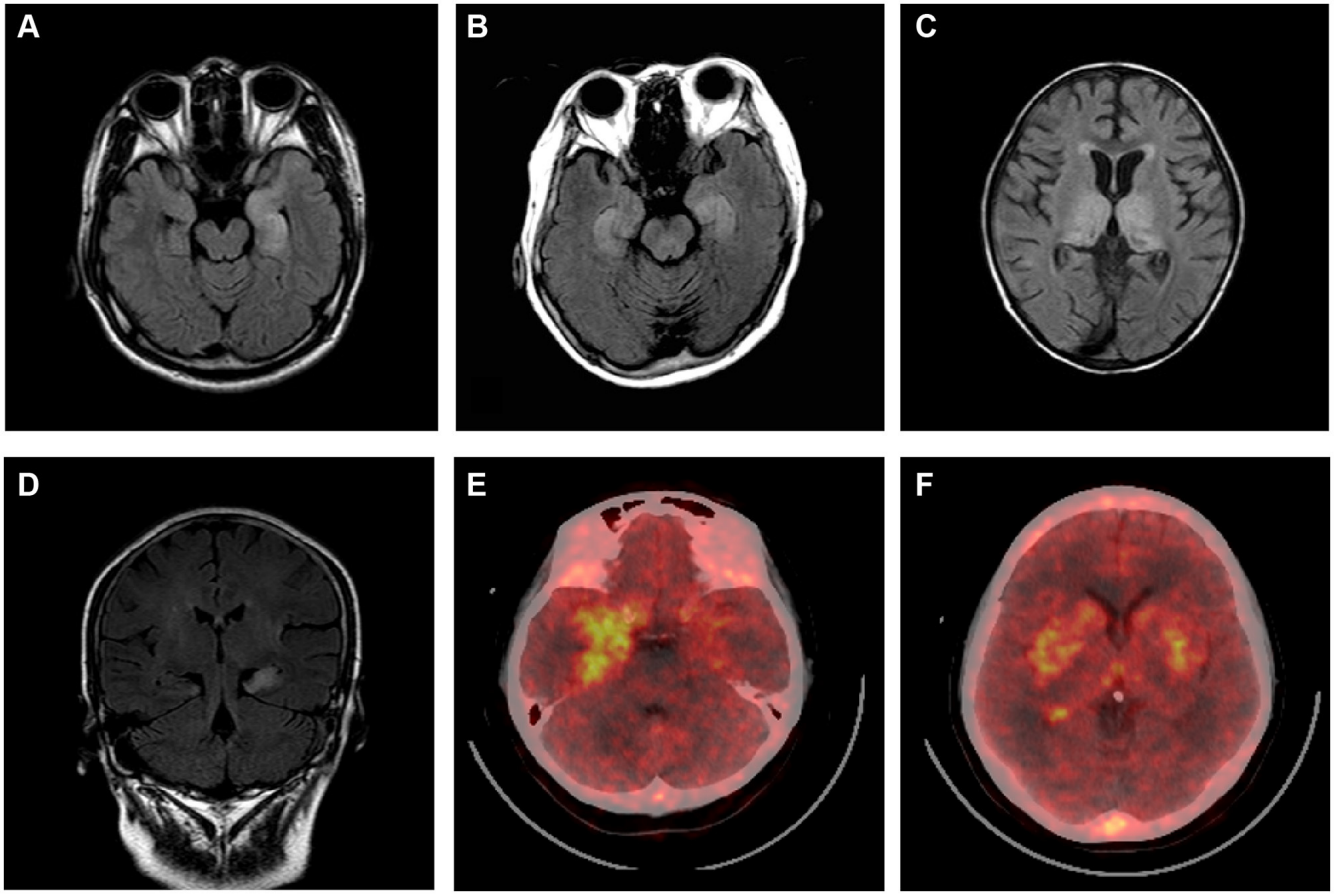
Brain MRI and positron emission tomography (PET) scans of patients with autoimmune encephalitis. Brain MRI T2-flair images (A–D). Bilateral high signal in the temporal lobe (A,B,D). Bilateral abnormal signals in the medial temporal lobe and occipital lobe (C). PET images show temporal cortex (more significant on the right) (E) and bilateral hypermetabolism in the basal ganglia (F).
